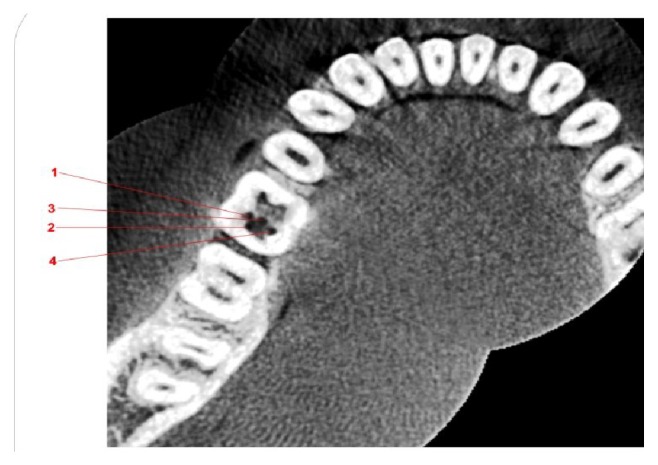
Enlarged CBCT axial section at the coronal one-third showing four distal and two mesial root canals, 1-distobuccal I, 2-distobuccal II, 3-middistal, and 4-distolingual.
